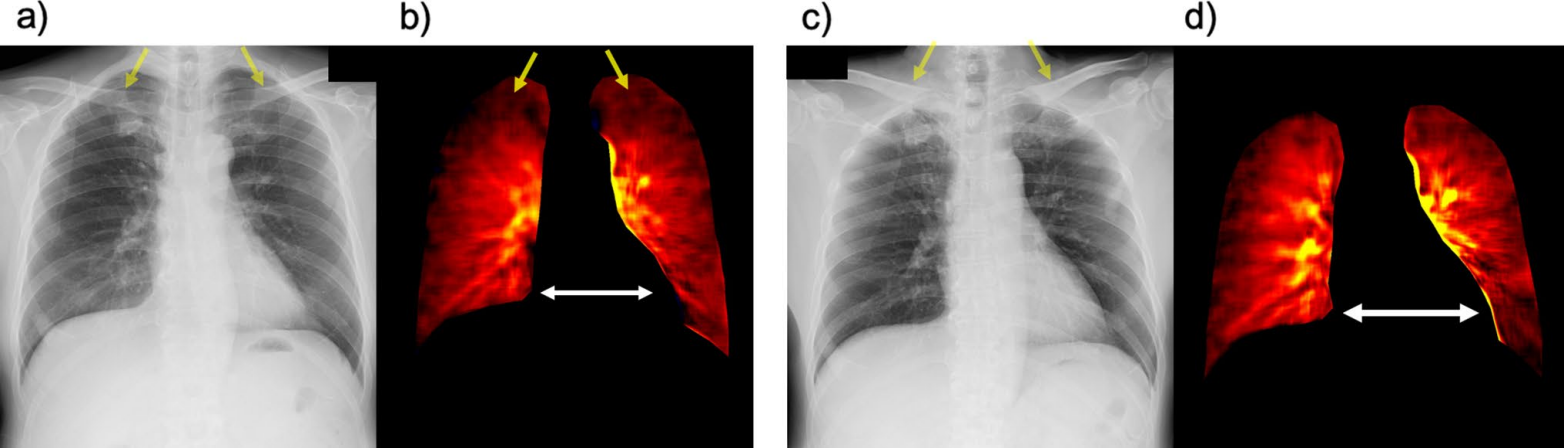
Images of a 66-year-old man without lung diseases. Clavicles superimposed on bilateral upper lungs on the chest radiograph captured in standing position (a, arrows), leading to a slight decrease in pulmonary perfusion in the dynamic perfusion image (b, arrows). In contrast, the clavicles shift upward in the chest radiograph captured in the supine position (c, arrows) so that pulmonary perfusion in the upper lungs is clearly visible (d). Furthermore, the heart shadow in the dynamic perfusion image captured in the supine position (d, two-headed arrows) is larger than that captured in the standing position (b, two-headed arrows), which may have led to decreased lung perfusion in the left lower lung
